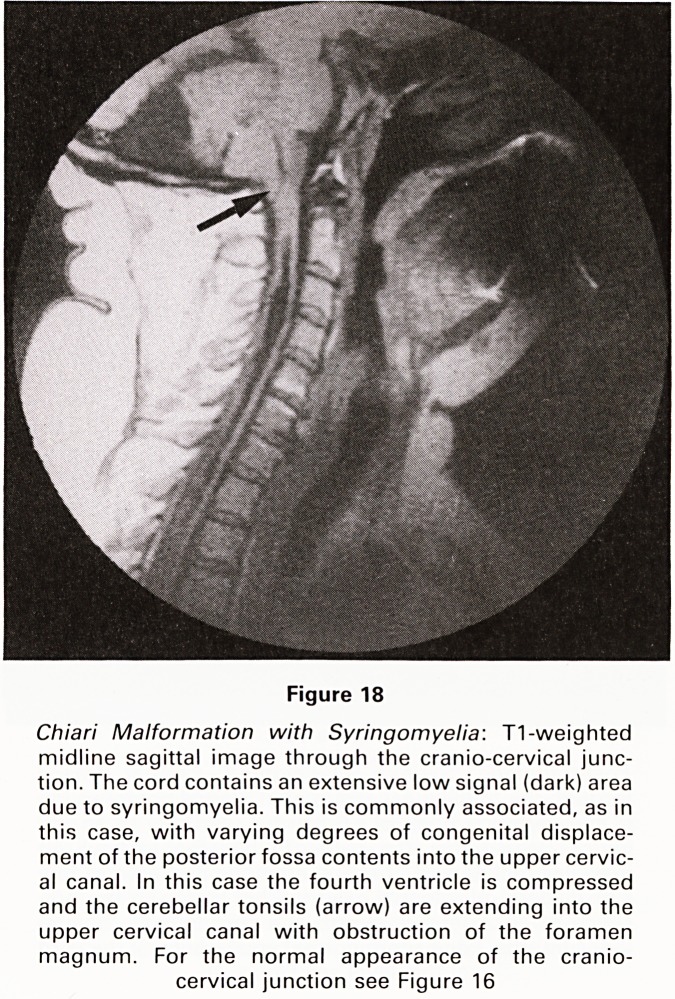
# Magnetic Resonance Imaging of the CNS
